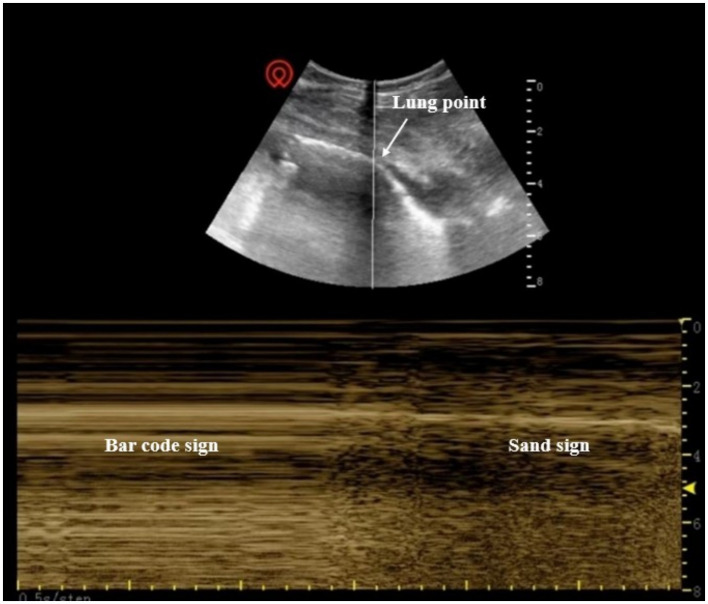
Pneumothorax on ultrasonography. Disappearance of the ultrasonographic “sand sign,” appearance of the “bar code sign,” or visible “lung point”.
